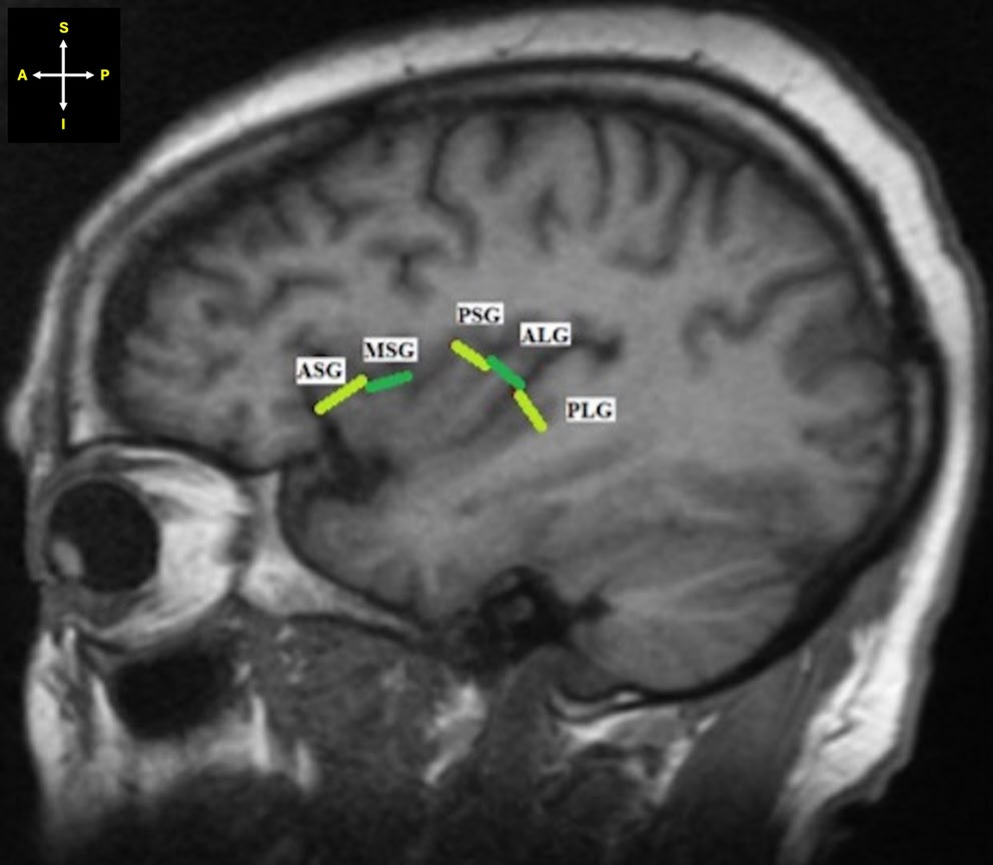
Sagittal section of MRI showing the maximum width of each gyrus. *Key* ASG -Anterior short insular gyrus; MSG - Middle short insular gyrus; PSG - Posterior short insular gyrus; ALG - Anterior long insular gyrus; PLG - Posterior long insular gyrus
